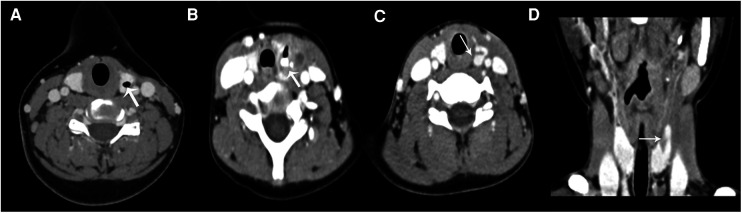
(A) A 12-year-old boy had a left neck mass for 8 years. Contrast-enhanced CT showed the local gas accumulation in the left lobe of the thyroid gland (arrow). (B) A 4-year-old boy had a recurrent infection in the left neck for more than 20 days. The thyroid showed local gas accumulation and high-density contrast medium residue (arrow); adjacent soft tissue swelling showed a low-density shadow. (C) (axial) and (D) (coronal). A 7-year-old boy had a left neck mass for 8 months. The contrast-enhanced CT showed a linear low-density shadow in the upper pole of the left lobe of the thyroid gland (arrow).
